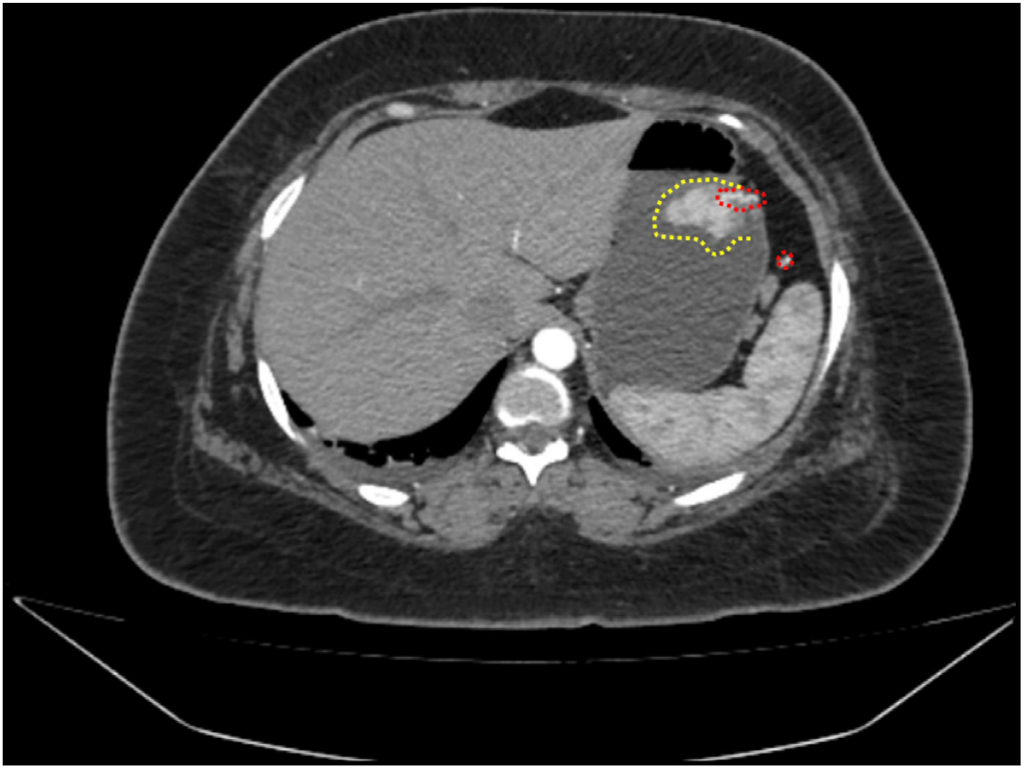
Contrast-enhanced computed tomography of the abdomen shows a large arterial enhancing lesion (*yellow dotted line*) in the body of the stomach along the greater curvature with feeder vessel arising from the short gastric branch (*red dotted line*).
